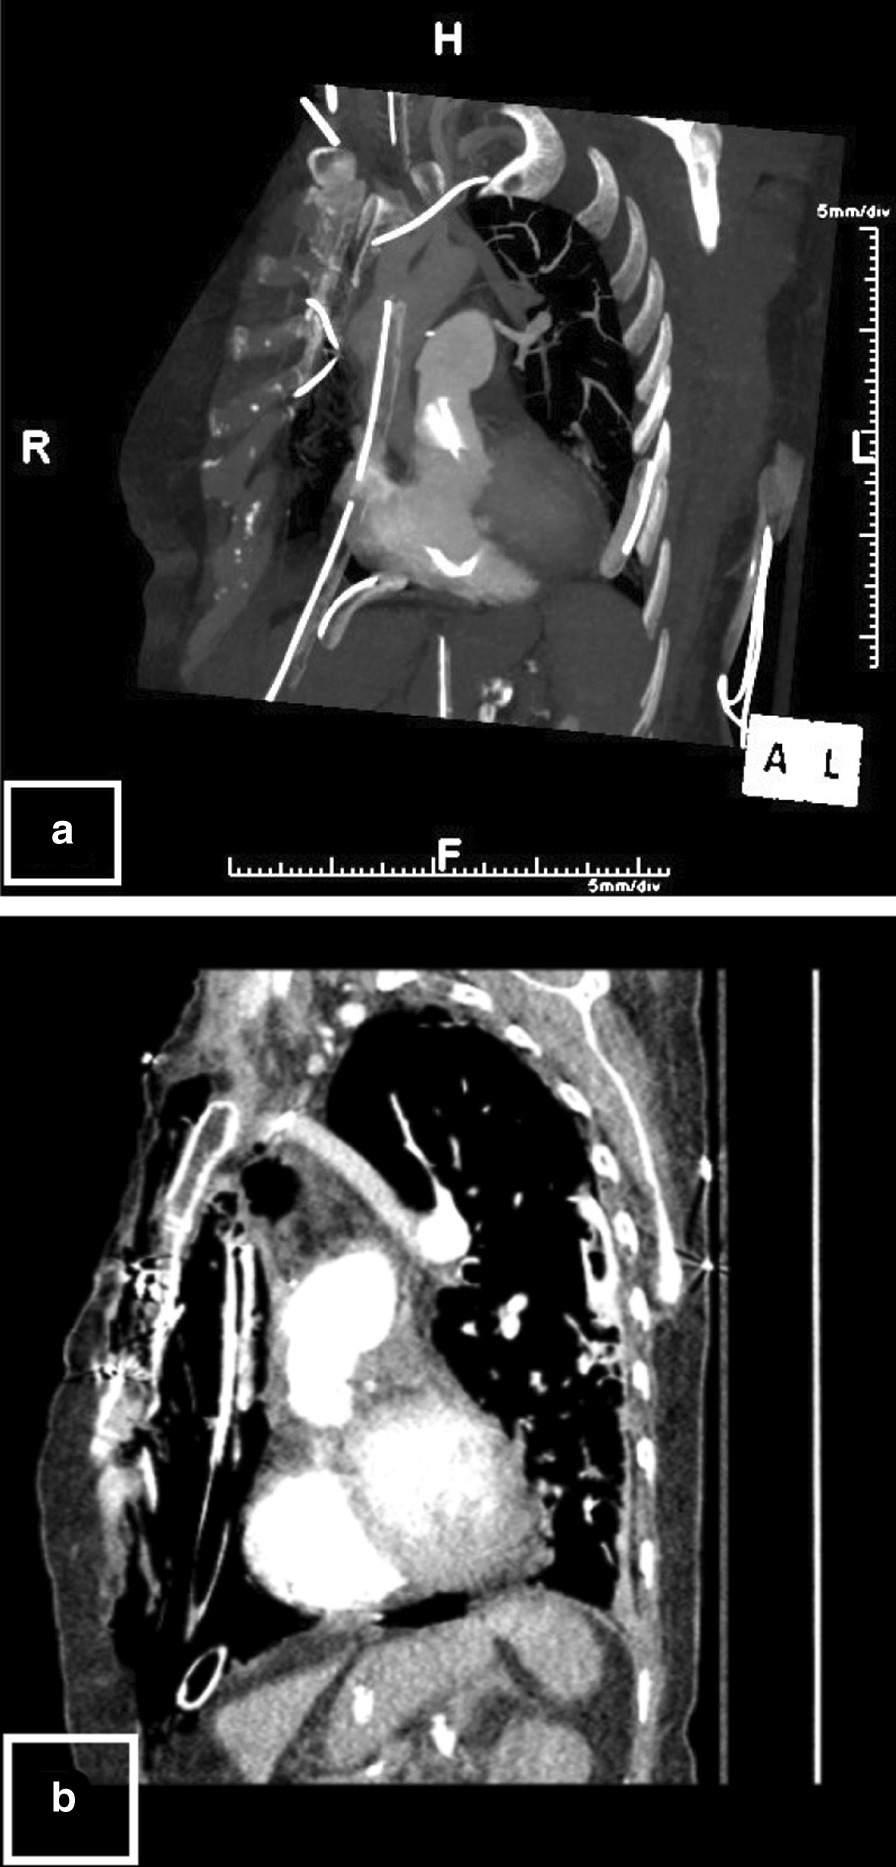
a The computed tomography (CT) image (sagittal view) shows the left superior pulmonary vein draining into the innominate vein. b CT Image with an alternate contrasting
